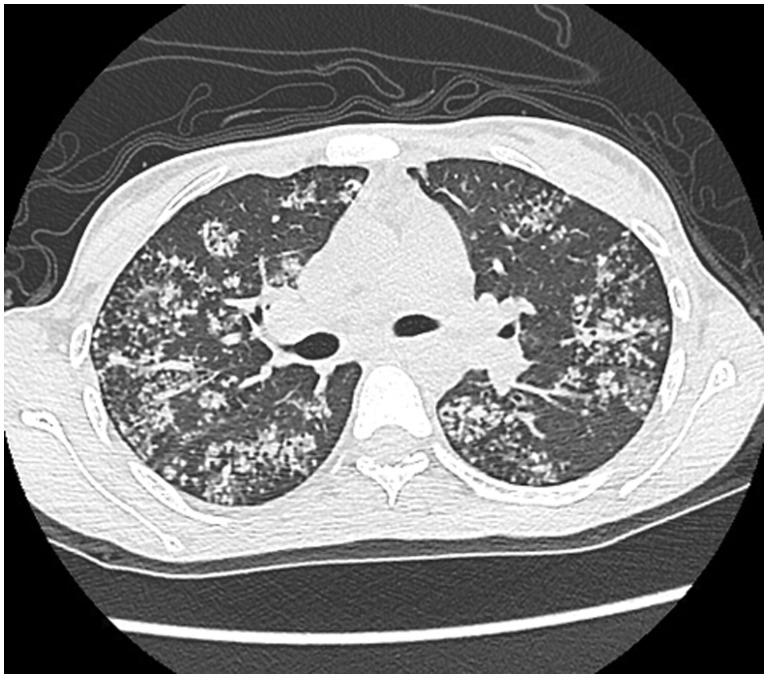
Pulmonary tuberculosis. High-resolution CT scan of lung window showing pulmonary tuberculosis in a case of cirrhosis. Centrilobular nodules are seen in both lungs with tree-in-bud appearance.
